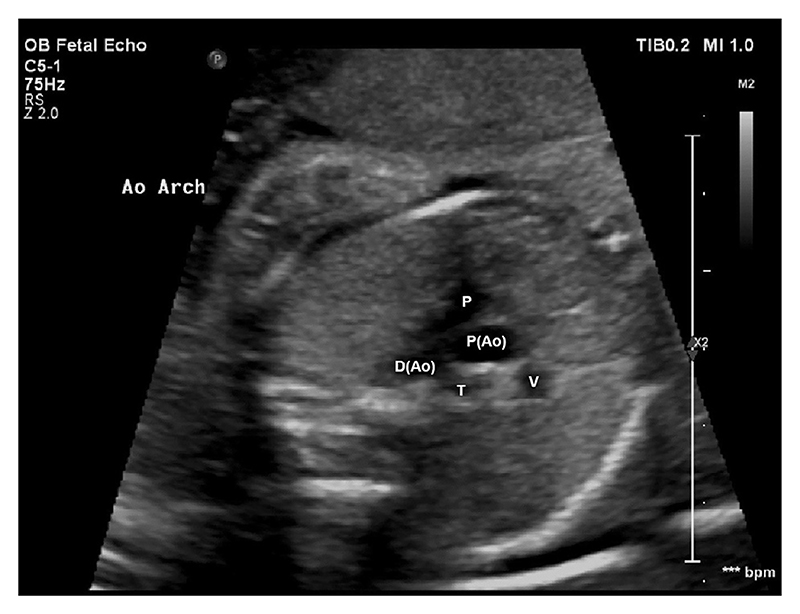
Obstetric ultrasound of fetal thorax in the 3-vessel-trachea view (3VT). Pulmonary artery (P), proximal aorta (P(Ao)), distal aorta (D(Ao)), superior vena cava (V), trachea (T).
